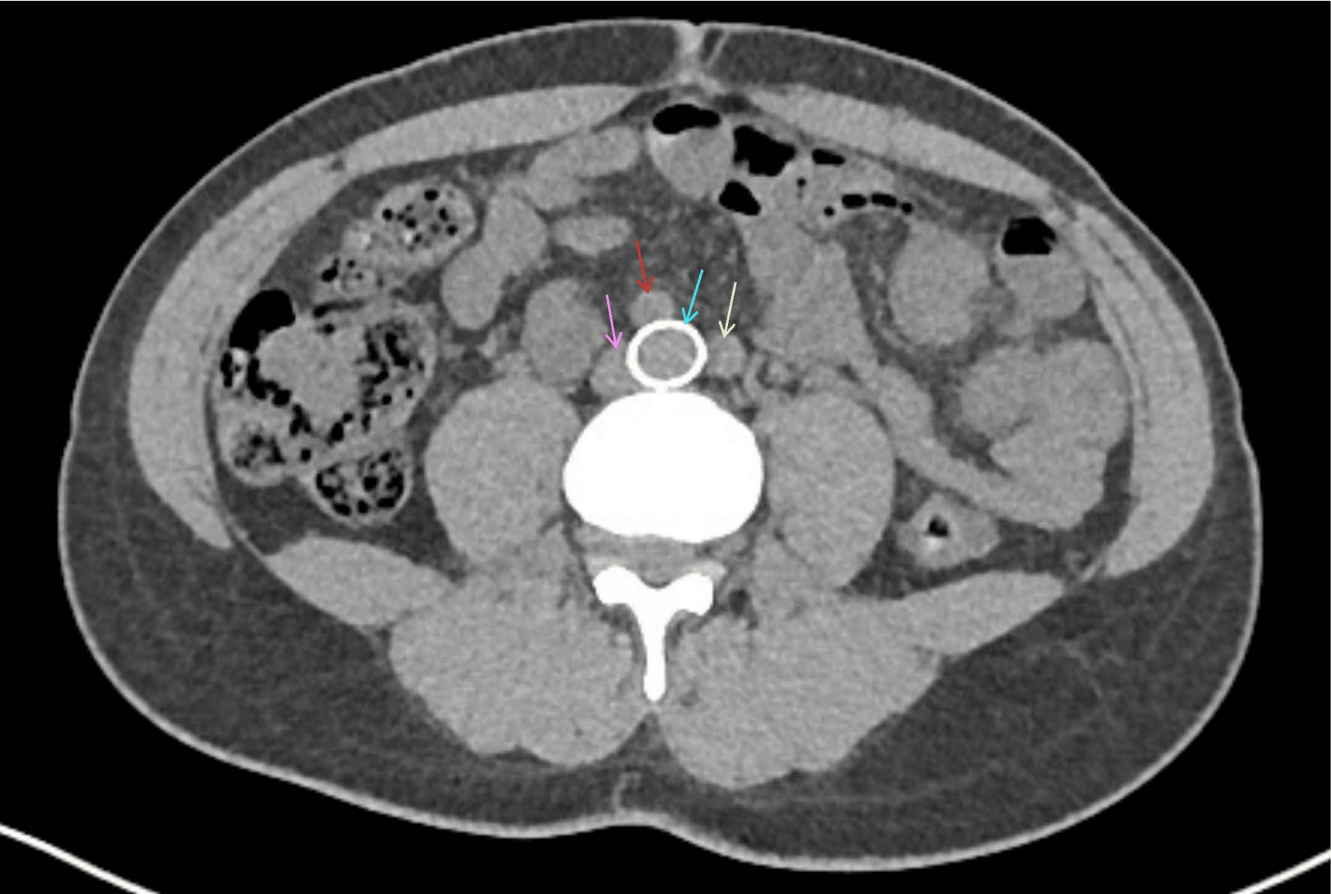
Axial CT view of the RCIA, LCIA, RCIV, and stented LCIV. CT: computed tomography; RCIA: right common iliac artery; LCIA: left common iliac artery; RCIV: right common iliac vein; LCIV: left common iliac vein The pink arrow shows the RCIV. The red arrow shows the RCIA. The blue arrow shows the stented LCIV. The yellow arrow shows the LCIA.
